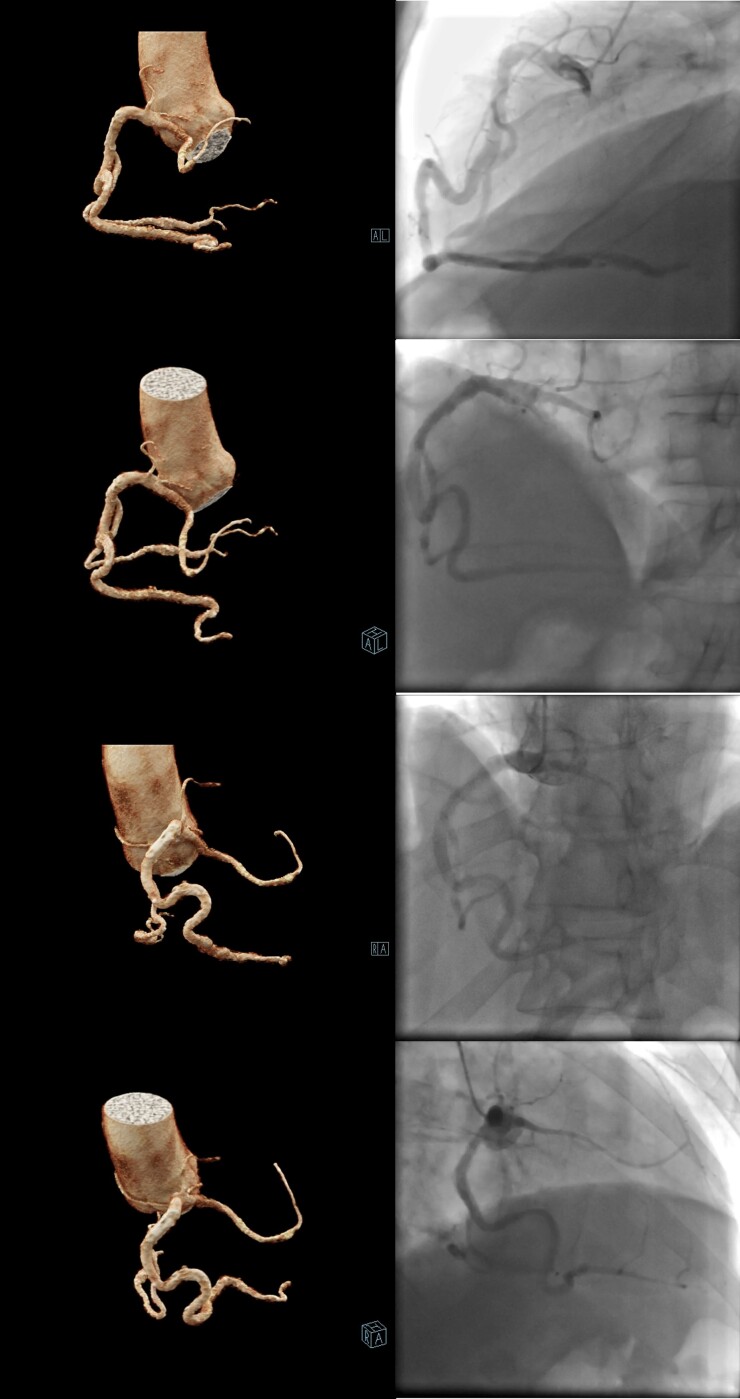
Invasive coronary angiography and computed tomography angiography images—the course of single right coronary artery.
